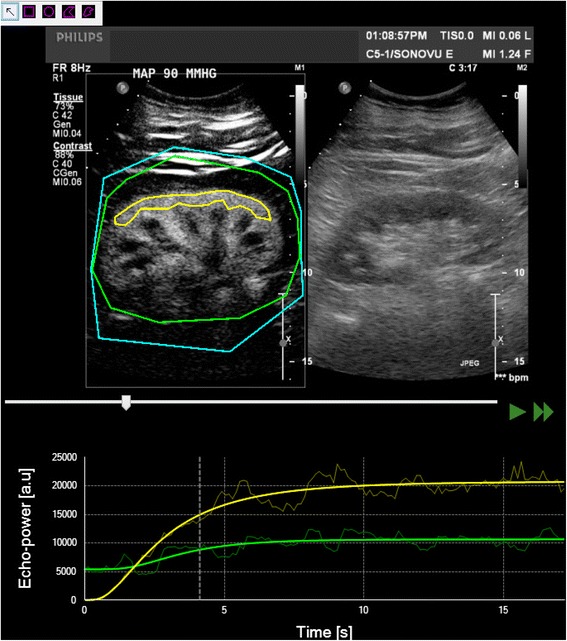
Sequence analysis with Vuebox®. A region of interest was drawn (yellow line) in the largest possible area of renal cortex close to the ultrasound probe. The software generates a time intensity curve (in yellow in lower panel). This curve is used to generate contrast-enhanced ultrasound (CEUS)-derived parameters. The green curve corresponds to the overall zone (kidney and surrounding tissues) and is not relevant for analysis. T0 corresponds to the destruction of all microbubbles in the scan plan by an ultrasound flash (increase of ultrasound intensity see text for details). Top right panel shows standard B-mode ultrasound image.
